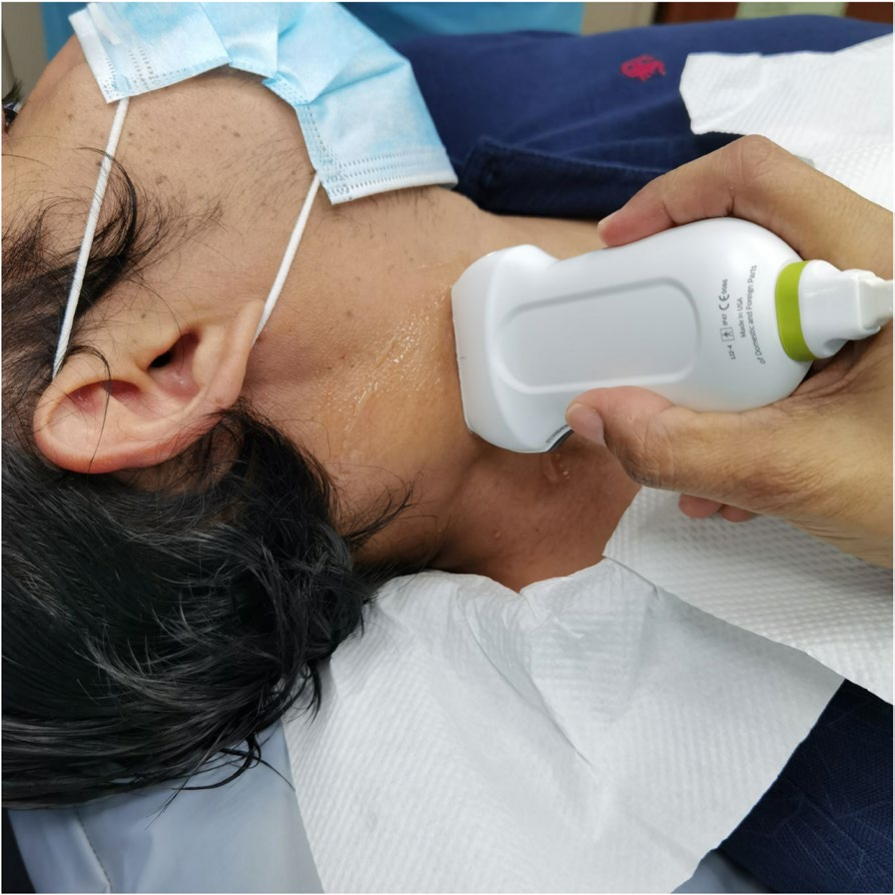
Surgeon performing USG using portable Philip system high-resolution ultrasound with broadband linear array
